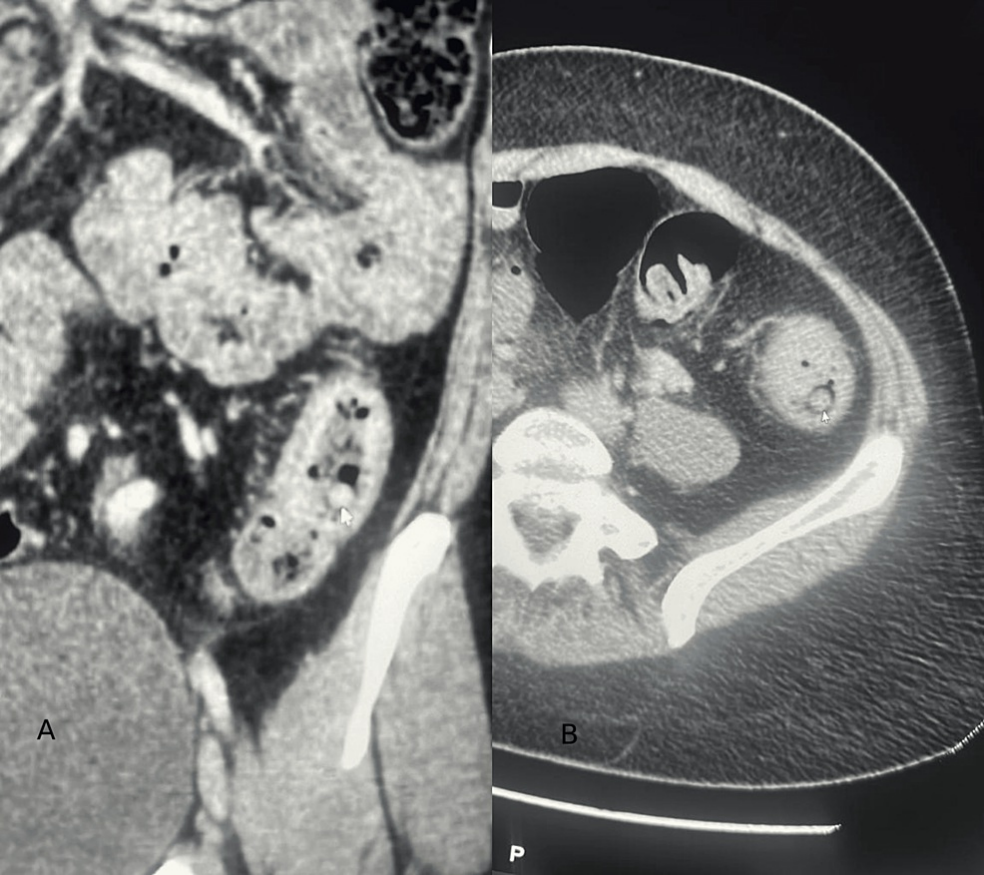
CT scan showing a focal circumferential proximal sigmoid wall thickening spanning 5.2 cm with a maximum thickness of 0.8 cm. It is associated with surrounding fat stranding and congested paracolic vessels.
